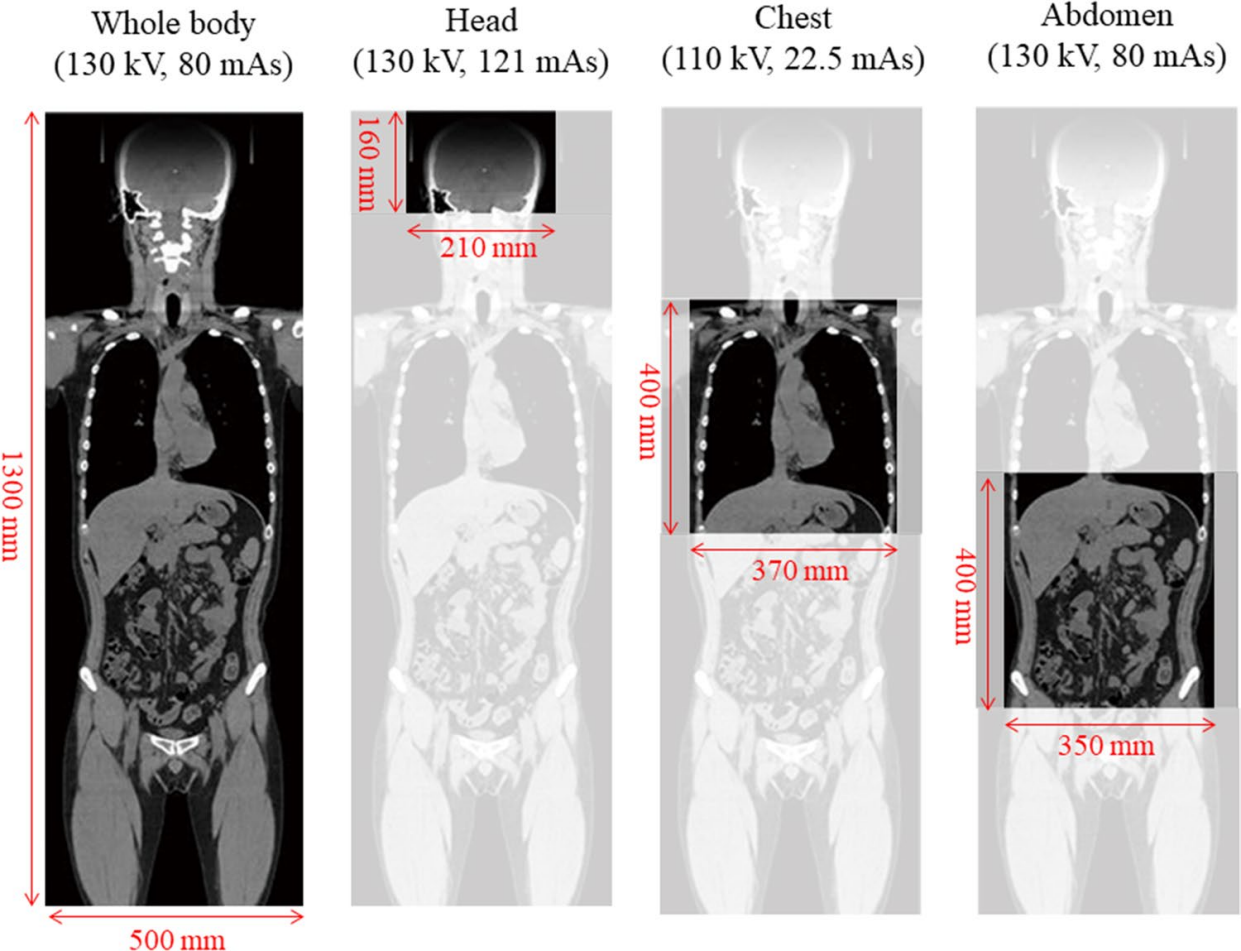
CT examination procedure and irradiation conditions. All examinations were performed using routine clinical protocols.
